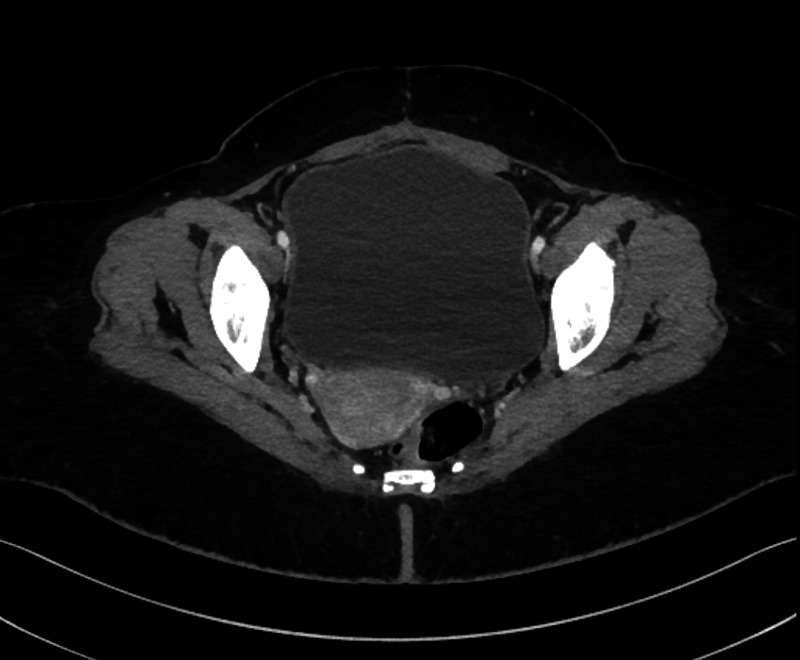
CT abdomen and pelvis with IV contrast at one and half year follow-up.
